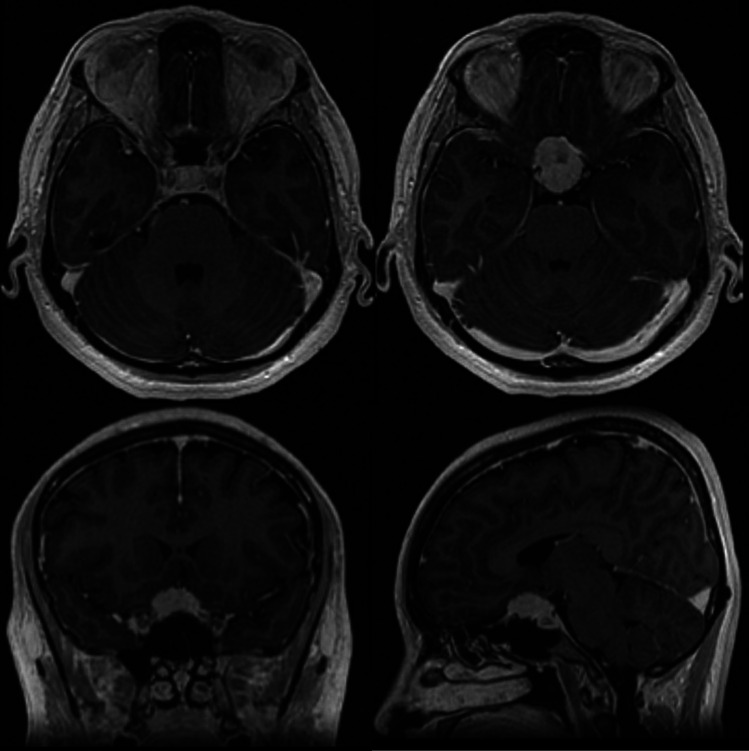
Preoperative MRI of a case with a tuberculum sellae meningioma. No tumor infiltration of the optic canal is visible. However, intraoperatively, tumor invasion inside the optic canal was found
